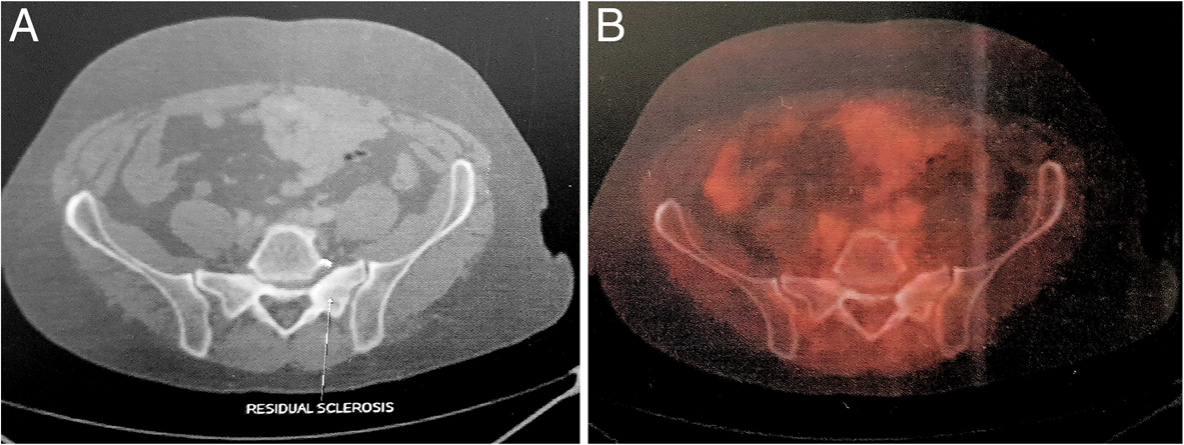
FDG-PET in 2017 after 6 cycles of R-CHOP regimen, external beam radiotherapy, and two cycles of maintenance rituximab. a Sclerosis at left ala of sacrum b with no metabolic uptake
